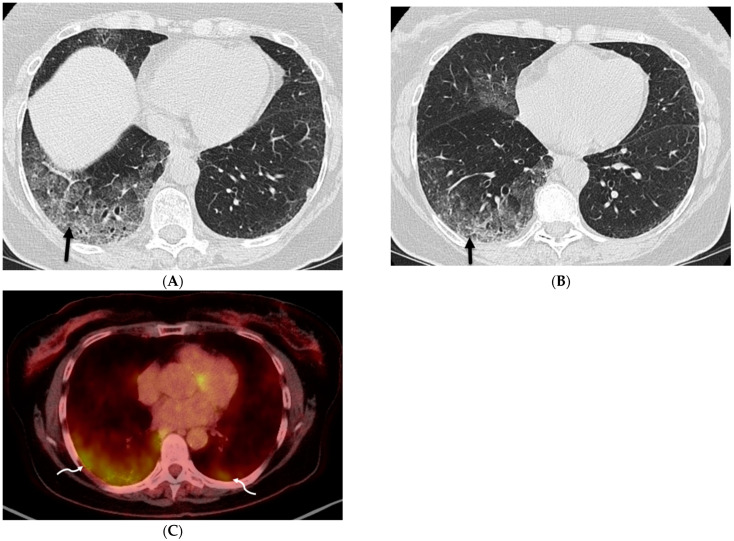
A 75-year-old former smoker presented with a history of chronic cough and was initially treated with antibiotics without improvement. Axial CT chest images obtained over a 4-month period (A,B) show worsening patchy ground glass opacity with areas of interlobular septal thickening (solid black arrow) in the right lower lobe. An axial fused FDG-PET/CT image (C) shows associated FDG uptake in lower lobes (curved white arrows), right greater than left, with a maximum standardized uptake value (SUV) of 5.4. Bronchoscopy confirmed the presence of multicentric invasive mucinous adenocarcinoma.
